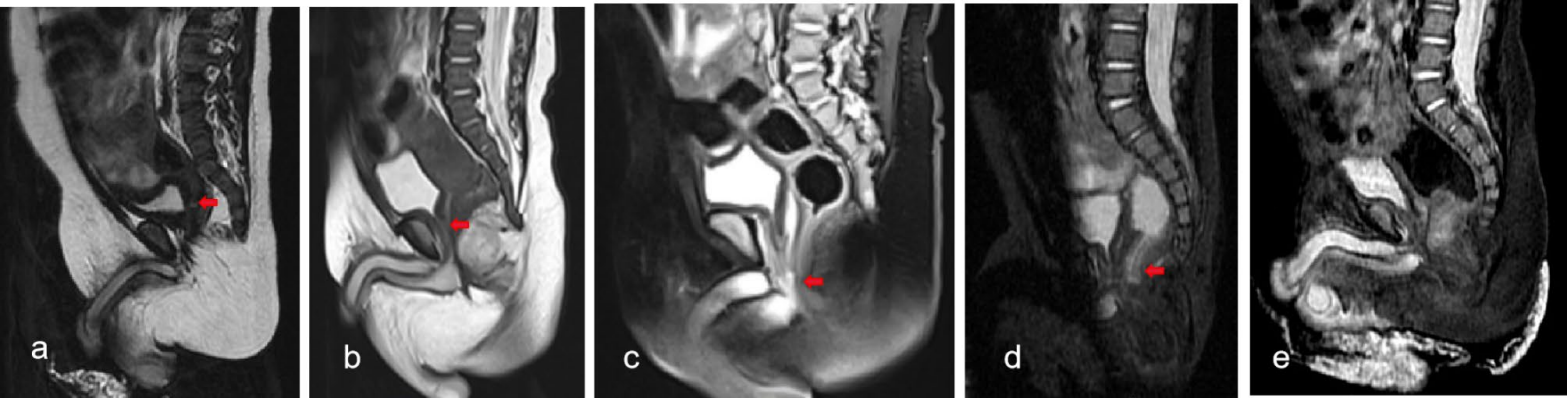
Fistula (red arrow) in MRI. (a) Rectovesical fistula. (b) Rectoprostatic fistula. (c) Rectobulbar fistula. (d) Fistula incompletely revealed. e.No fistula
